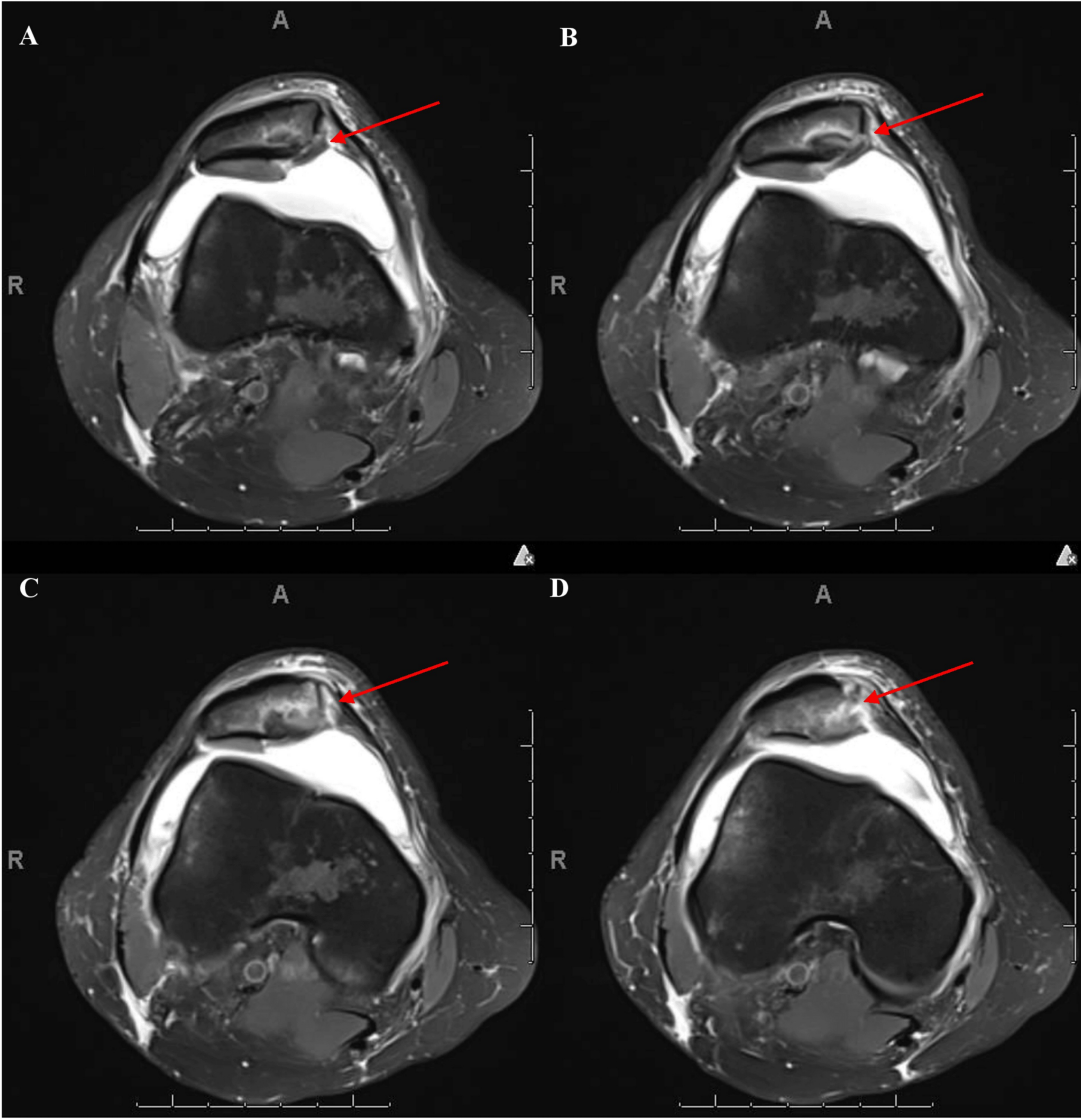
Pre-operative axial MRI images (A, B, C, D) demonstrate an osteochondral fragment of the posterior lateral femoral condyle embedded within the medial patellar facet - proximal inferior aspect with associated elevation of patella (A, B), and distal inferior aspect with (C) and without (D) evidence of healing. A: Pre-operative axial MRI image suggestive of osteochondral fragment of posterior lateral femoral condyle embedded inside of the proximal inferior aspect of the medial patellar facet and causing elevation. B: Pre-operative axial MRI demonstrating an osteochondral fragment from the posterior lateral femoral condyle, embedded within the middle inferior aspect of the medial patellar facet, resulting in elevation. C: Pre-operative axial MRI image suggestive of osteochondral fragment of posterior lateral femoral condyle embedded inside of the distal inferior aspect of the medial patellar facet with evidence of healing. D: Pre-operative axial MRI image suggestive of osteochondral fragment of posterior lateral femoral condyle embedded inside of the distal inferior aspect of the medial patellar facet. Red arrows in all the figures indicate the osteochondral fragment of posterior lateral femoral condyle embedded inside of the inferior aspect of the medial patellar facet.
